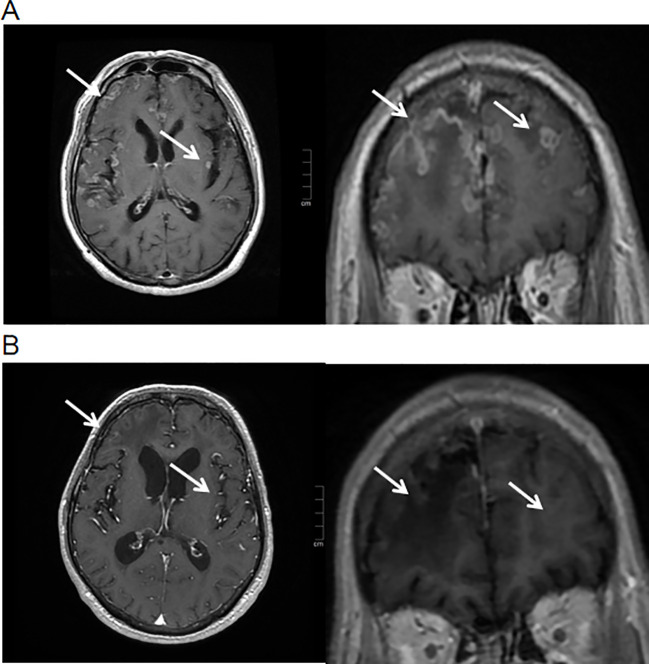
Head contrast-enhanced MRI. (A) This showed the nodular and ring enhancement of the fronto-temporo-parietal lobes before savolitinib treatment (white arrow). (B) MRI profile 8 months post-savolitinib treatment (white arrow).
